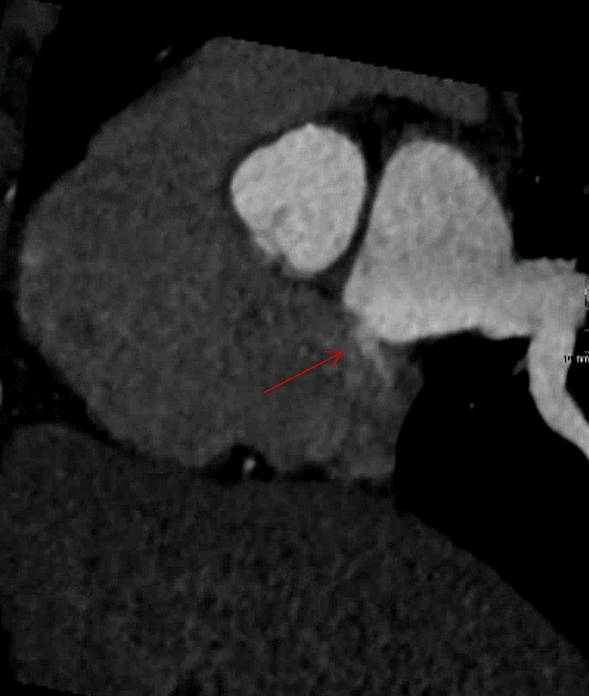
Cardiac CT showing a left-to-right contrast jet (red arrow) scored as PFO. TTE with agitated saline contrast did not detect a PFO in this patient
